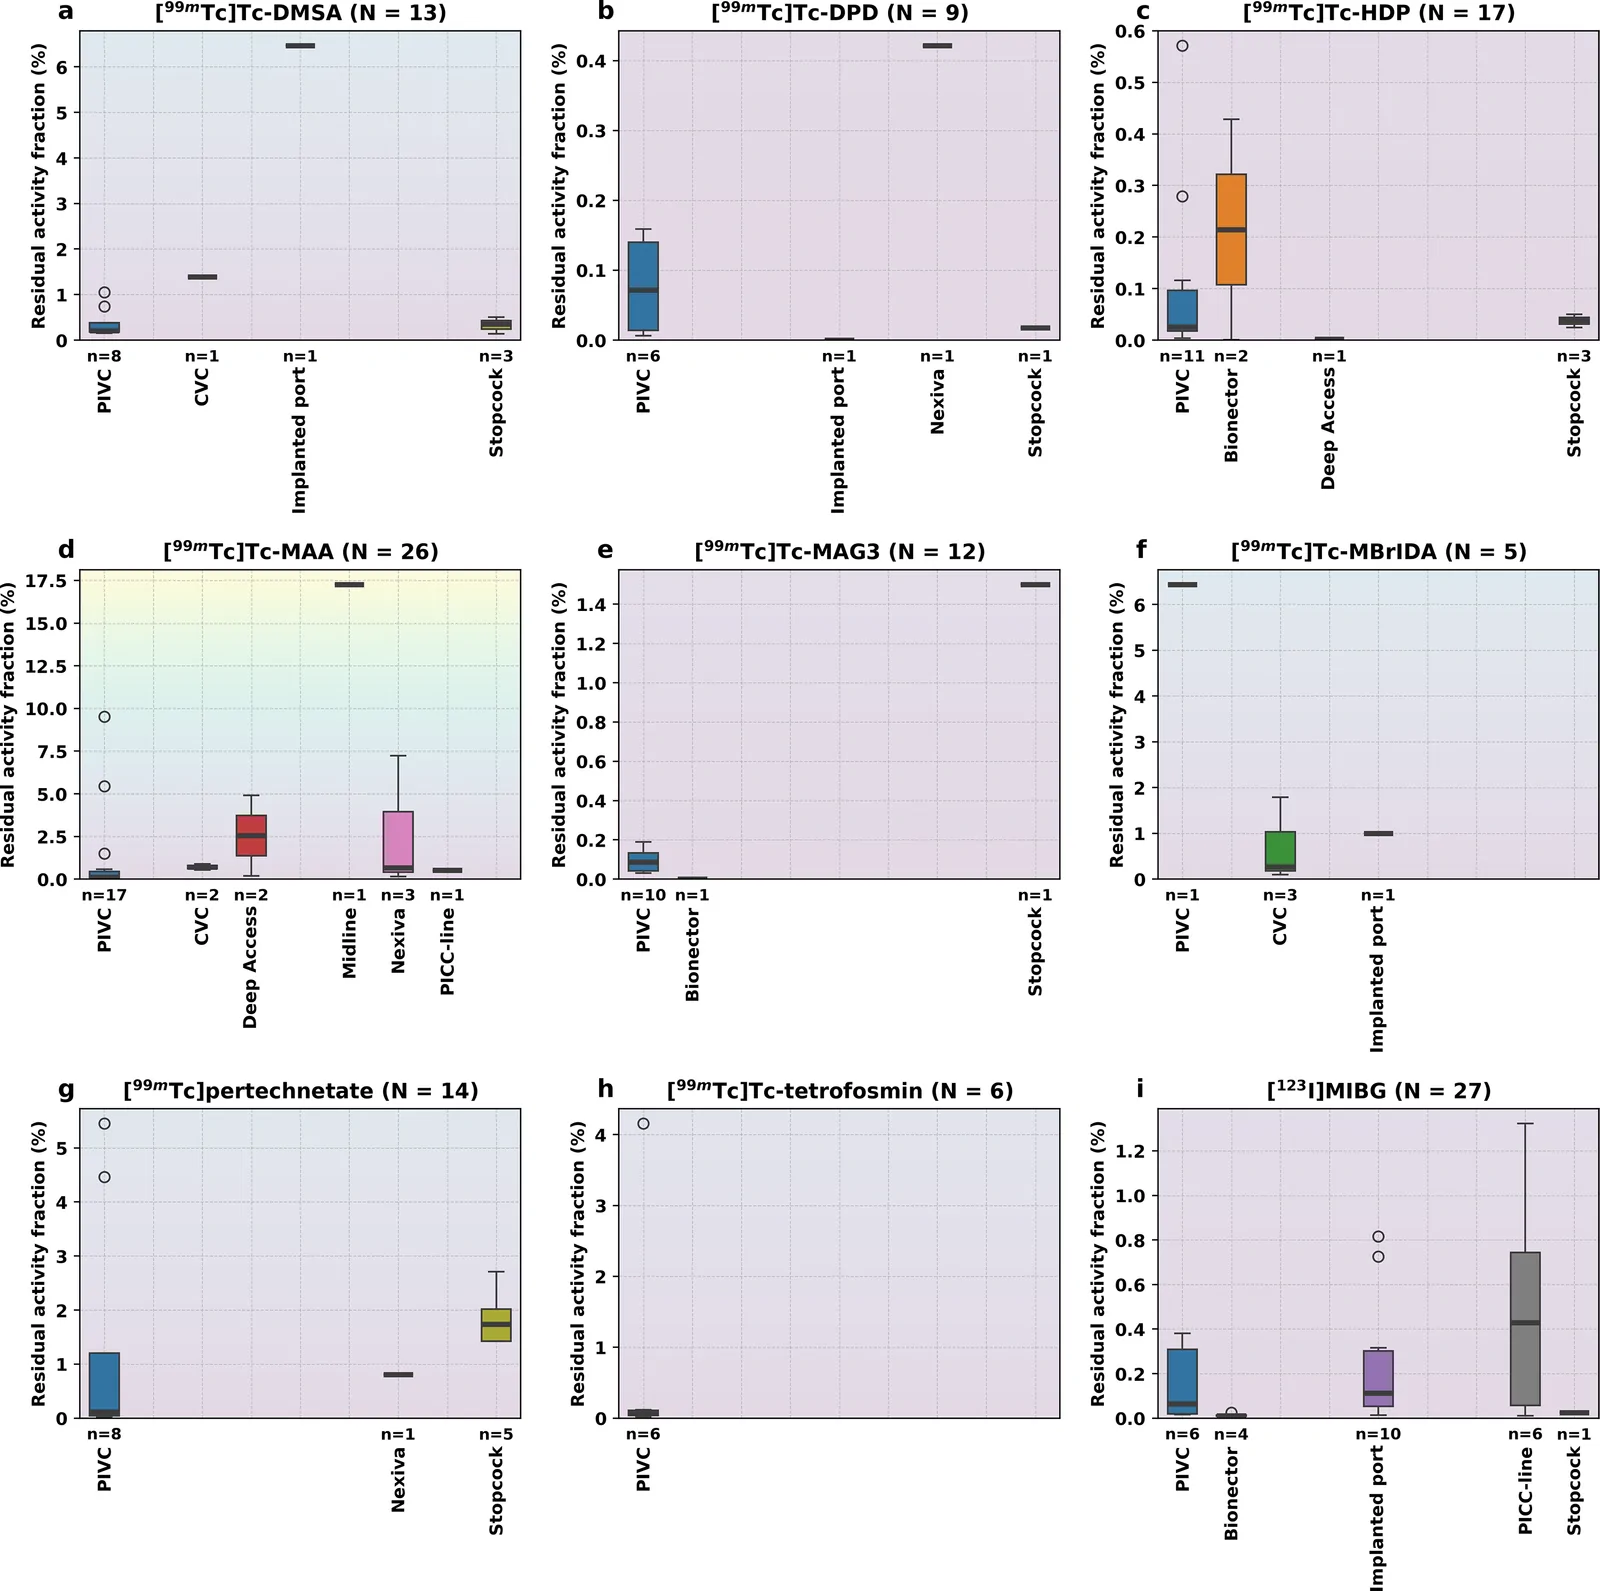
Residual activity fraction for the SPECT radiopharmaceuticals (a-i) for patients administrations. Note the different range of the y-axes of the sublots, highlighted by the color gradient background ranging from zero to the global maximum of the figure
